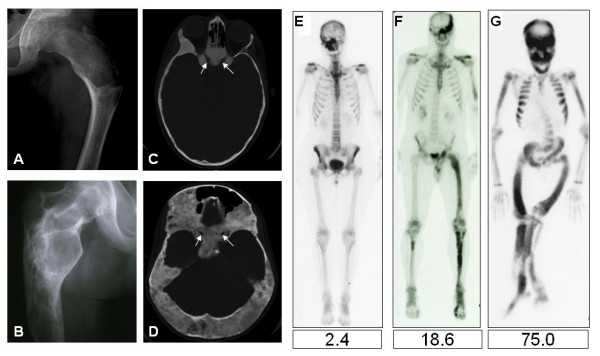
Radiographic appearance of fibrous dysplasia (FD). A) A proximal femur with typical ground glass appearance and shepherd's crook deformity in a 10-year-old child is shown. B) The appearance of FD in the femur of an untreated 40-year-old man demonstrates the tendency for FD to appear more sclerotic with time C) The typical ground glass appearance of FD in the craniofacial region on a CT image of a 10-year-old child is shown. The white arrows indicate the optic nerves, which are typically encased with FD. D) A CT image in a 40-year-old woman demonstrates the typical appearance of craniofacial FD in an older person, with mixed solid and "cystic" lesions. The Hounsfield Unit measurements of "cystic" lesions are quite useful in distinguishing soft tissue "cystic" lesions from true fluid-filled cysts, which are much more uncommon and tend to behave aggressively with rapid expansion and compression of vital structures. E-G) Bone Scintigraphy in FD. Representative ^99^Tc-MDP bone scans which show tracer uptake at affected skeletal sites, and the associated skeletal disease burden score (see ref. Collins, 2005) are shown. E) A 50-year-old woman with monostotic FD confined to a single focus involving contiguous bones in the craniofacial region. F) A 42-year-old man with polyostotic FD shows the tendency for FD to be predominantly (but not exclusively) unilateral, and to involve the skull base and proximal femur. G) A 16-year-old boy with McCune-Albright syndrome and involvement of virtually all skeletal sites (panostotic) is shown [65].
